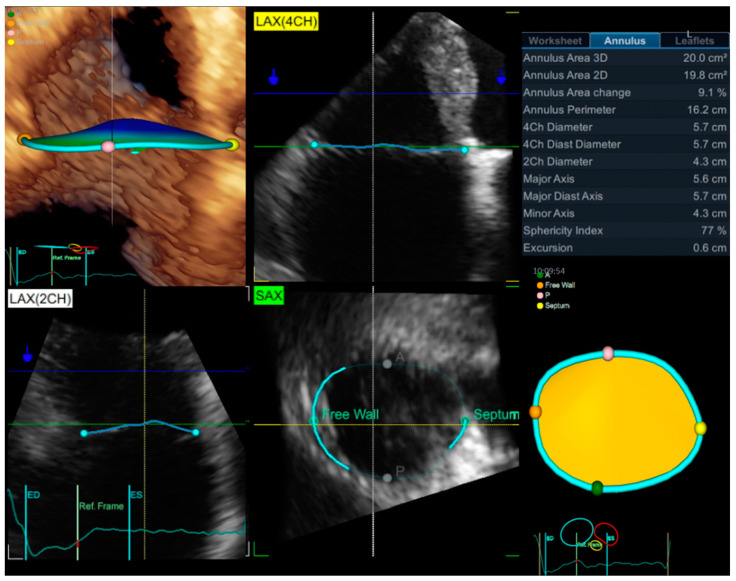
Evaluation of tricuspid annular size and shape by 4D Auto TVQ software ((EchoPac v204, GE, Horten, Norway). Abbreviations: 2Ch, apical 2-chamber view; 2D, two-dimensional echocardiography; 3D, three-dimensional echocardiography; 4Ch, apical 4-chamber view; A, anterior; ED, end-diastole; ES, end-systole; LAX, long axis; P, posterior; SAX, short axis.
